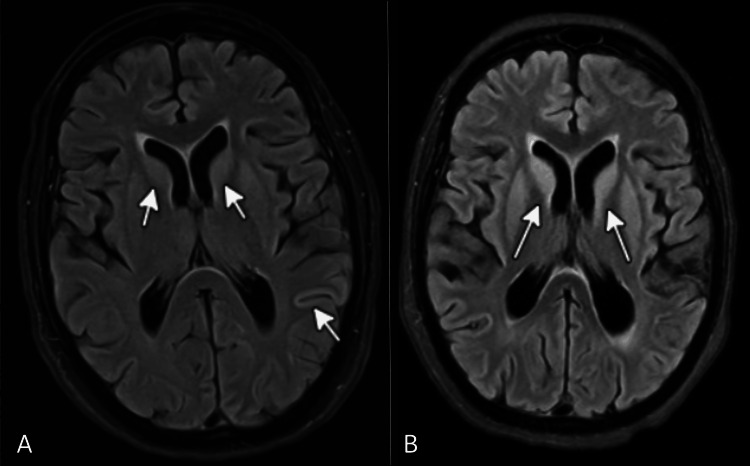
Serial Brain MRI Demonstrating Progressive Changes in Creutzfeldt-Jakob Disease (CJD) (A) Initial brain MRI showing diffusion-weighted imaging (DWI) hyperintensities involving the bilateral caudate nuclei and subcortical cortical ribboning in the insular and parietal regions, suggestive of early prion disease (white arrows). (B) Follow-up brain MRI performed 57 days later reveals the progression of DWI hyperintensities involving the bilateral caudate nuclei, putamen, insular cortices, posterior temporal lobe cortices, and medial frontal lobes, consistent with advancing CJD (white arrows).
